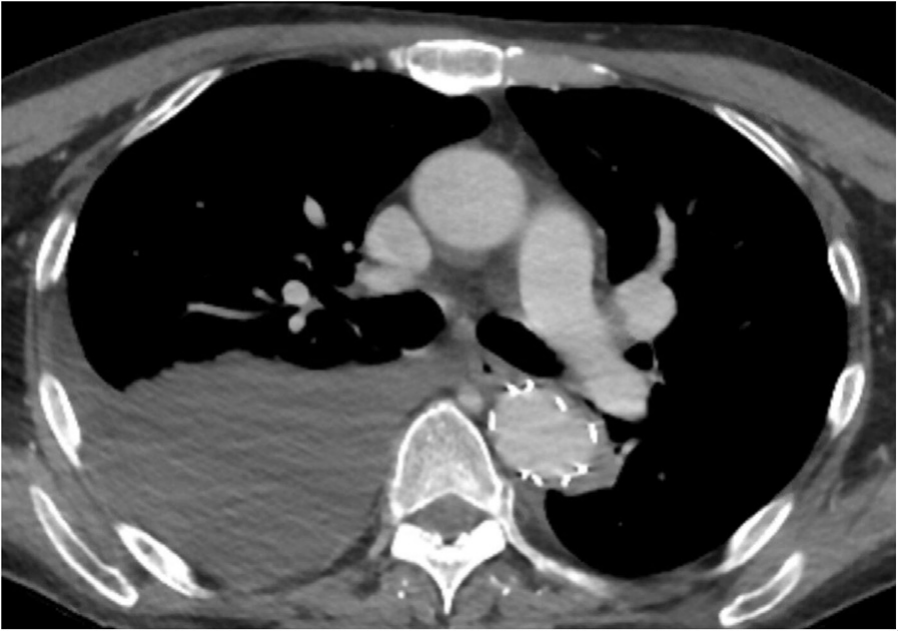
Computed tomography angiography (CTA) displaying axial view after thoracic endograft deployment showing successful exclusion of the saccular mycotic aneurysm and no evidence of an endoleak.
